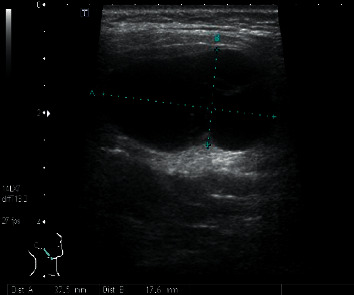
Ultrasonography of the right parotid gland revealed a 37.5 × 17.6 × 24.2 mm multilocular cystic lesion with clearly defined margins.
